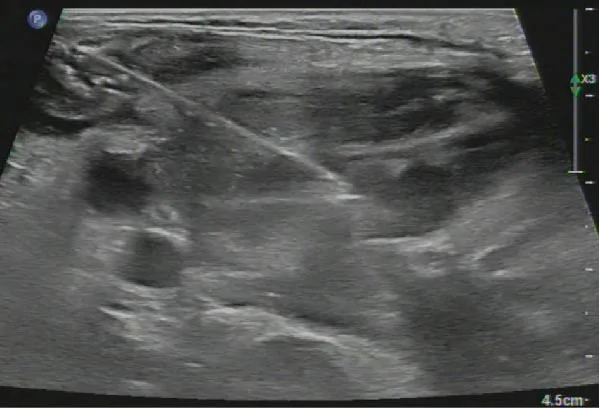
Ultrasound‐guided drainage via thyroid catheterization.
